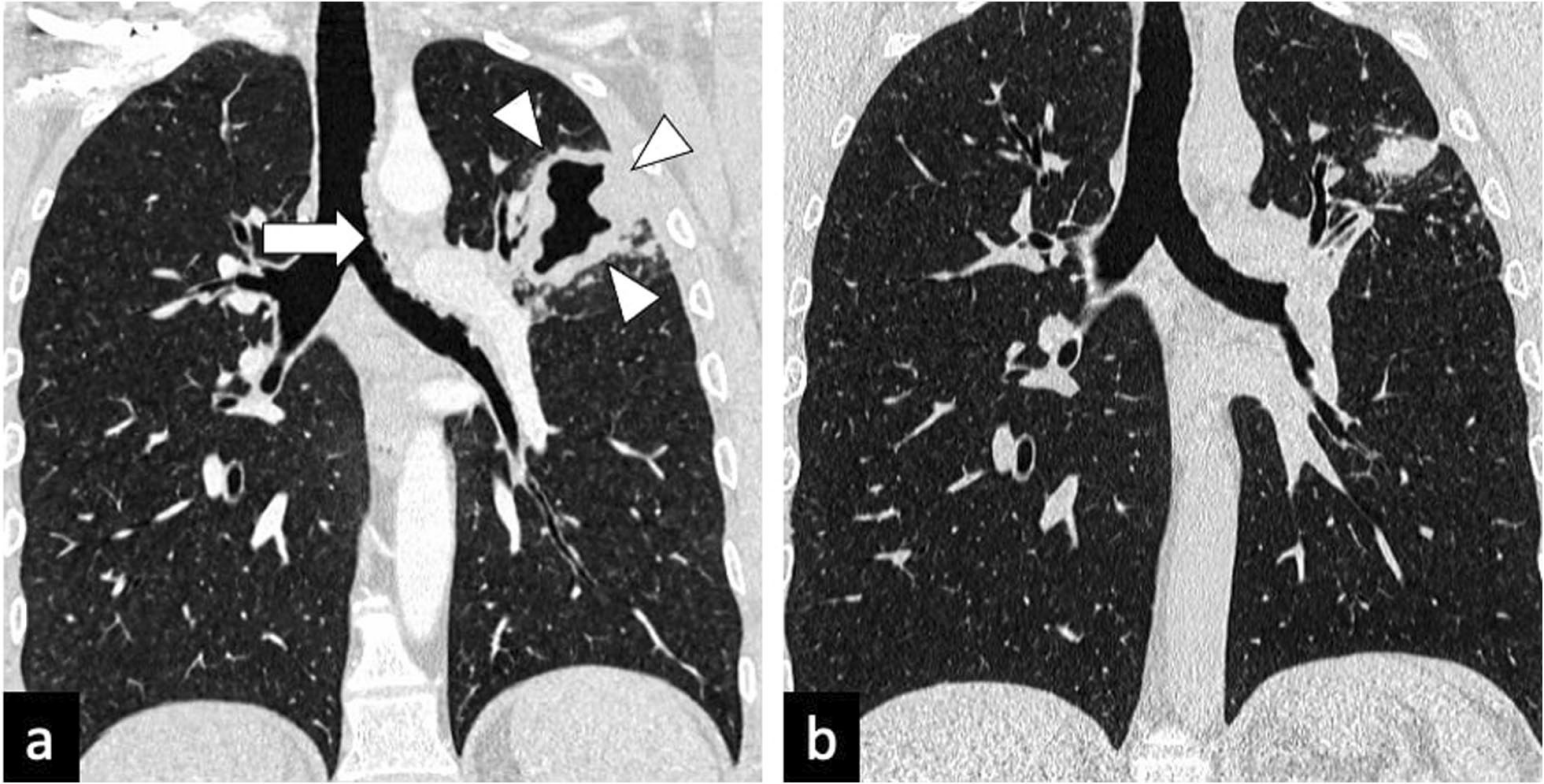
A 35-year-old male presented with a 4-week history of productive cough, anorexia and weight loss. Initial CT demonstrates tracheal and left main bronchus wall thickening with irregularity suggestive of ulcerations (lung window, coronal plane—a, arrow). There is also a thick-walled cavity with surrounding nodularity (arrowheads). The appearances significantly improved following TB treatment as demonstrated on a follow-up CT performed two months later (lung window, coronal plane—b)
